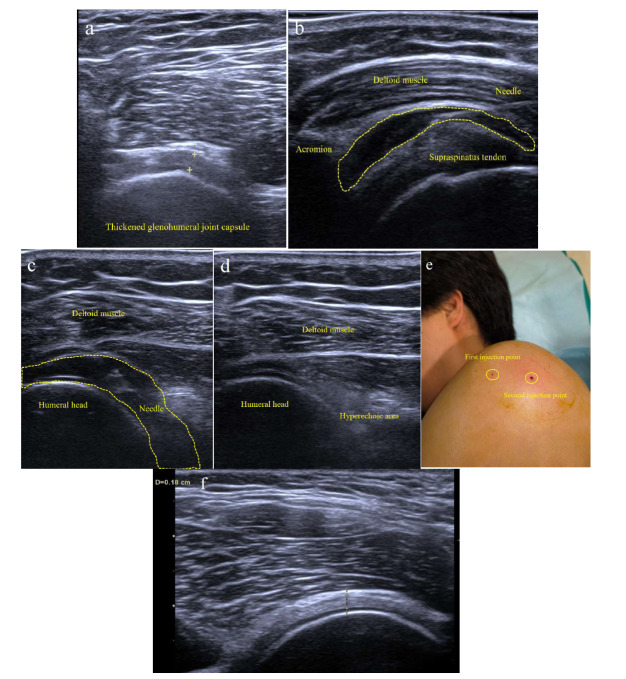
a) Ultrasound images for diagnosing FS. The glenohumeral joint capsule was significantly thickened (0.30cm). b) Subacromial bursa was widened after injection under US guidance (anechoic area rounded by yellow dotted line). c)Saline mixed with air bubbles were injected under US guidance in the posterior recess bursa of the glenohumeral joint to do hydrodilatation. Glenohumeral joint was widened (anechoic area rounded by yellow dotted line). d) Flaky hyperechoic area can be observed due to the reflection of air bubbles. e) After the treatment, only two tiny needle eyes can be seen. f)3 months after treatment, normal glenohumeral joint capsule (0.18cm).
